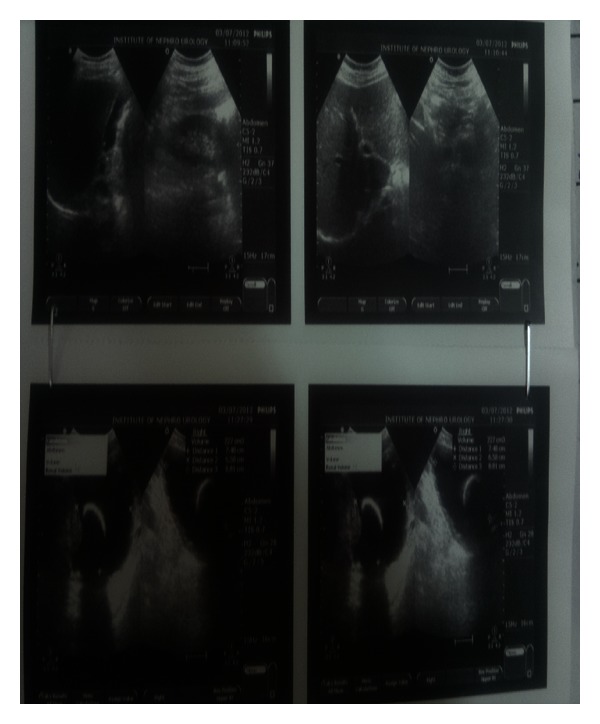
Ultrasonogram showing the giant calculus in bladder extending into urethra.
